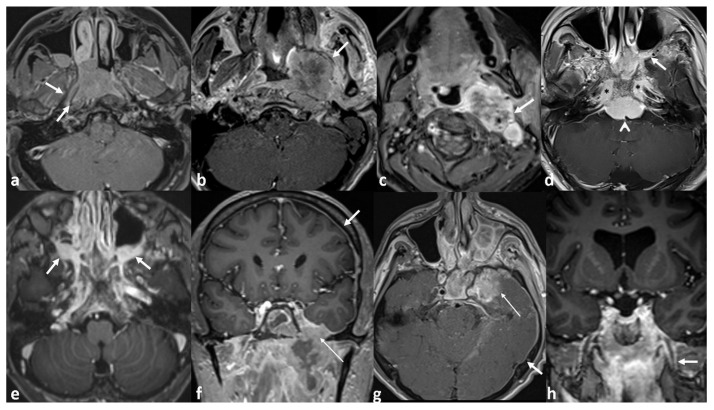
Post-contrast axial and coronal T1-weighted MR images showing patterns of tumor extension. (a) Tumor extends beyond the pharyngobasillary fascia but is confined to the tensor veli palatini. (b) Mass-forming left-sided tumor invading the lateral pterygoid muscle (arrow), indicating extended PPS invasion. (c) Extended PPS invasion with ICA encasement in the carotid space (arrow). (d) ICS and skull base invasion involving the foramen lacerum (stars), left pterygopalatine fossa (arrow), and prepontine cistern (arrowhead). (e) Infiltrative pattern with bilateral enlargement of pterygopalatine fossae. (f) CN V involvement with widening of the foramen ovale (thin arrow) and dural infiltration (thick arrow). (g) Parenchymal invasion of the medial temporal lobe, dural spread (arrows), and cavernous ICA invasion. (h) Perineural spread along CN V (arrow). CN, cranial nerve; ICA, internal carotid artery; PPS, parapharyngeal space; SB, skull base; ICS, intracranial space.
